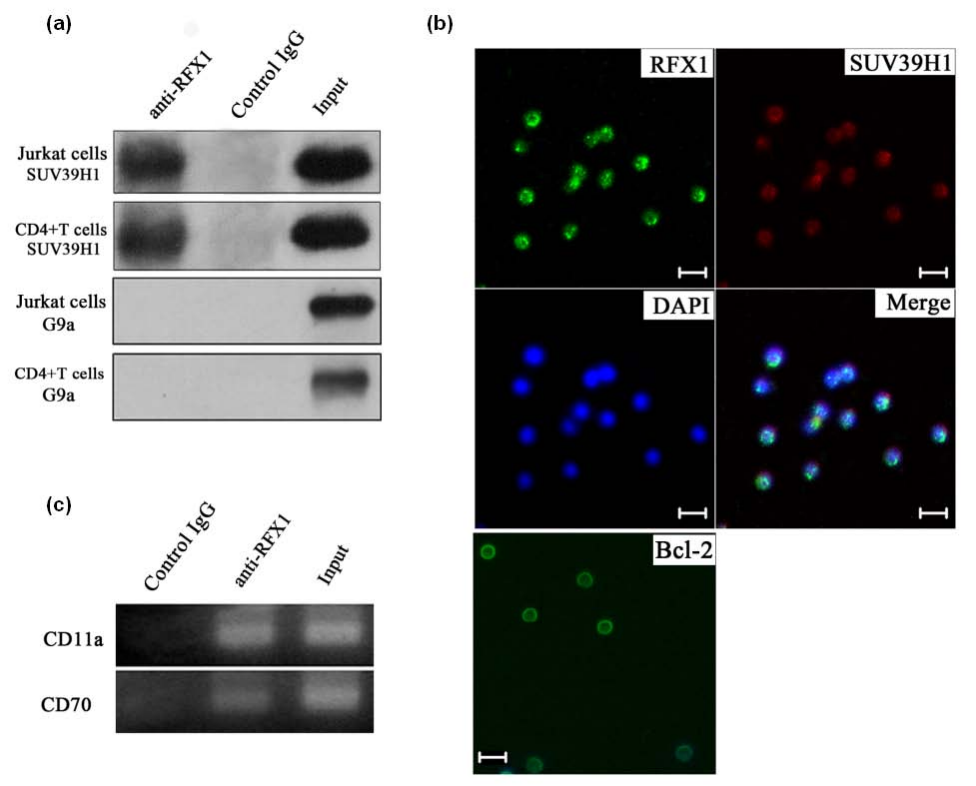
SUV39H1 physically interacts with RFX1, and is recruited to CD11a and CD70 promoters. (a) Anti-SUV39H1 western blots of Jurkat cells (top) and healthy CD4^+ ^T cells (bottom) lysates following immunoprecipitation with the antibodies indicated at the top. (b) CD4^+ ^T cells from healthy controls co-immunolabeled with anti-RFX1 (green) and anti-SUV39H1 (red). DAPI (blue) was used to label cell nuclei. Merged images are also shown. Anti-Bcl-2 antibody was included as control to cytoplasm of CD4^+ ^T cells (green). Scale bar, 10 μm. (c) Anti-SUV39H1 ChIP assay of CD4^+ ^T cell lysates. CD11a and CD70 promoters were identified by PCR amplification of the DNA fragments precipitated with SUV39H1 antibody.
